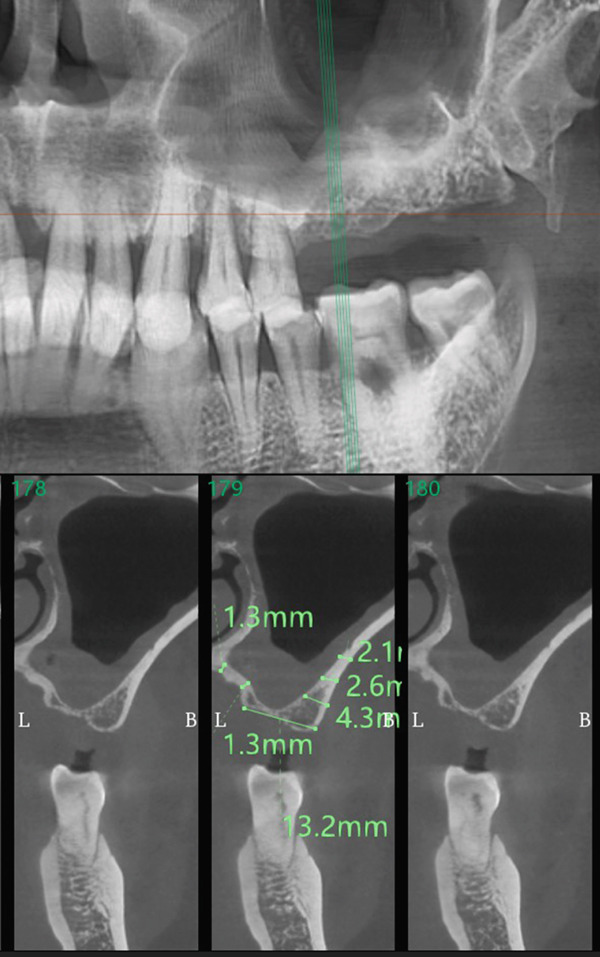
Preoperative CBCT scan of Tooth #26. The buccal sinus wall exhibits thicknesses of 4.3, 2.6, and 2.1 mm at the 3‐, 6‐, and 9‐mm levels, respectively. The palatal wall thicknesses at the 3‐ and 6‐mm levels are both 1.3 mm, and the width of the alveolar crest measures 13.2 mm.
